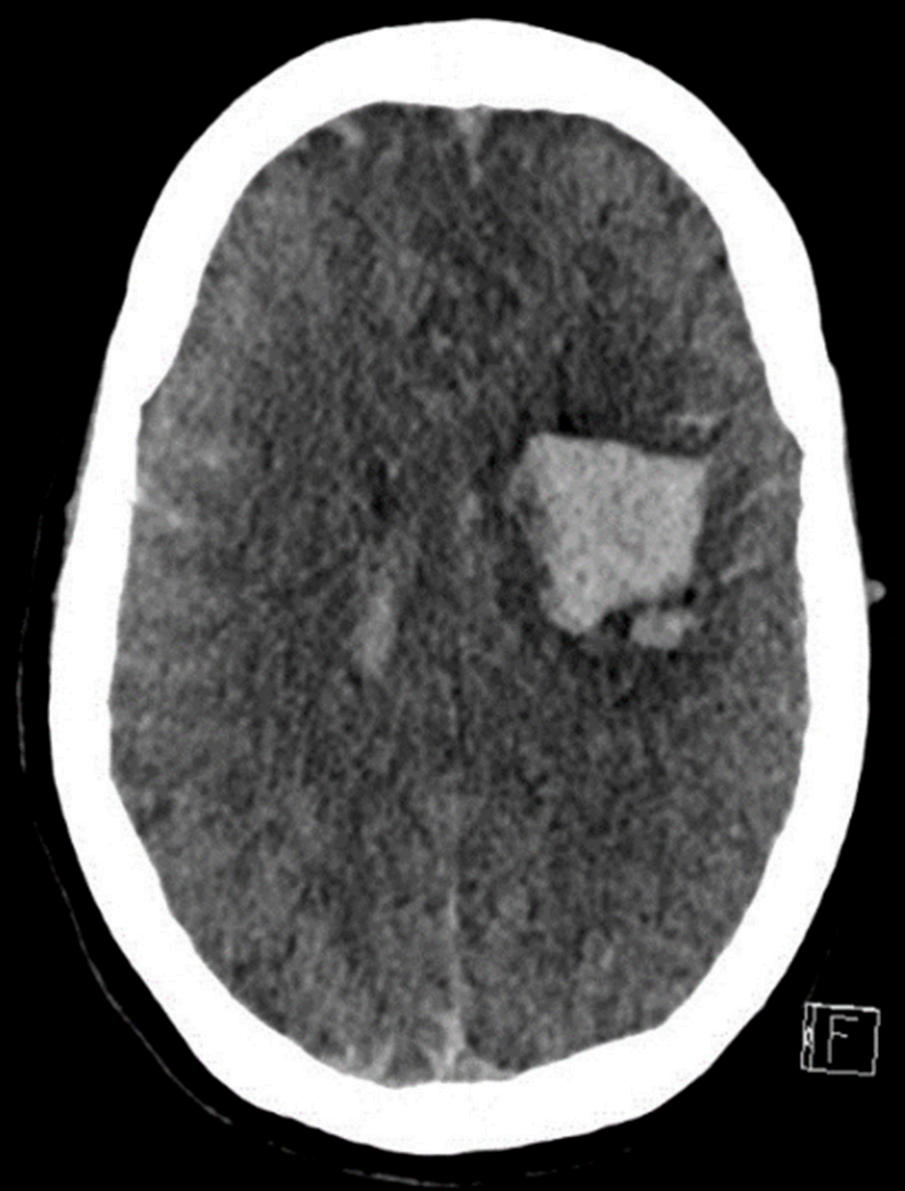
There is an acute left frontal parenchymal haemorrhage, which presents a similar distribution to the area of diffusion restriction identified on MRI in 2016.
